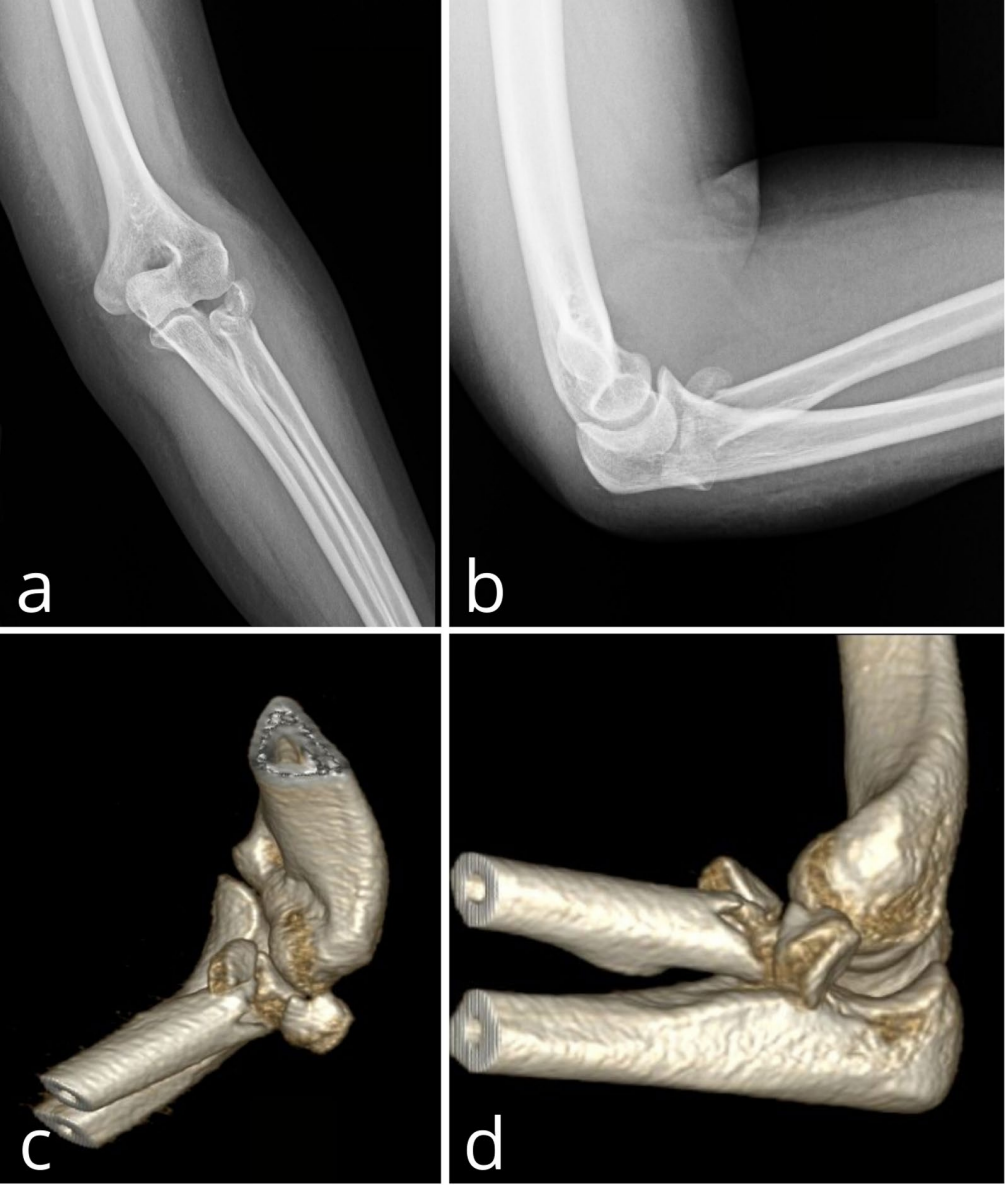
Anteroposterior (a) and lateral (b) X-ray and 3D CT (c, d) images of a patient with Mason type III radial head fracture
